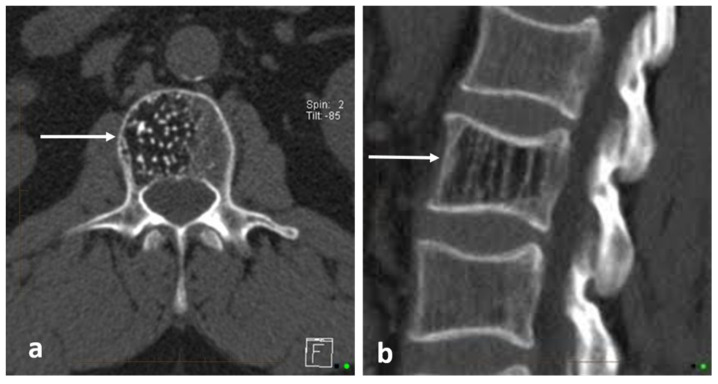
Axial (a) and sagittal (b) CT images demonstrating a lumbar vertebral haemangioma (white arrows). Note typical ‘polka dot’ appearance on axial image, and the ‘corduroy’ pattern on sagittal image.
